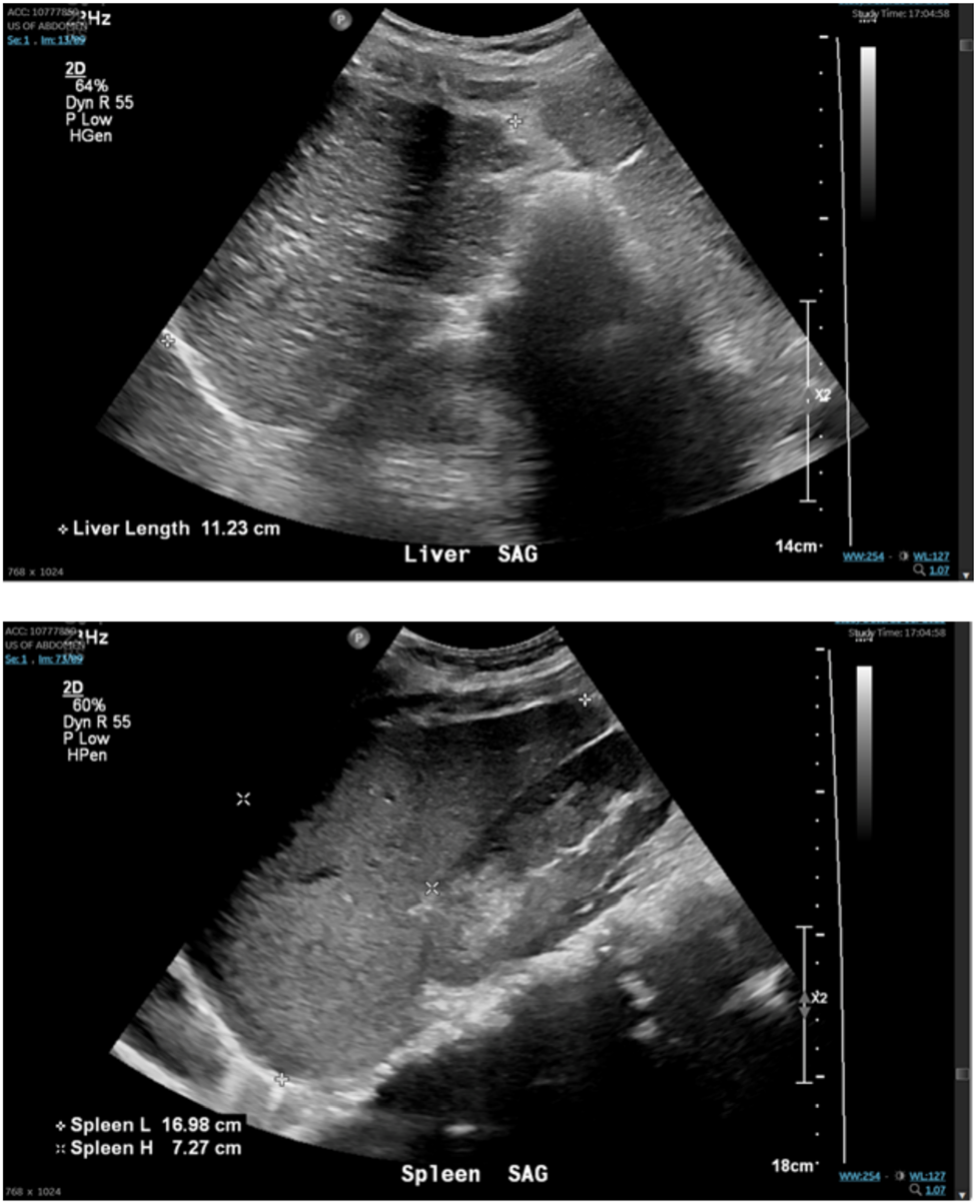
Abdominal ultrasound demonstrating liver cirrhosis and splenomegaly.
